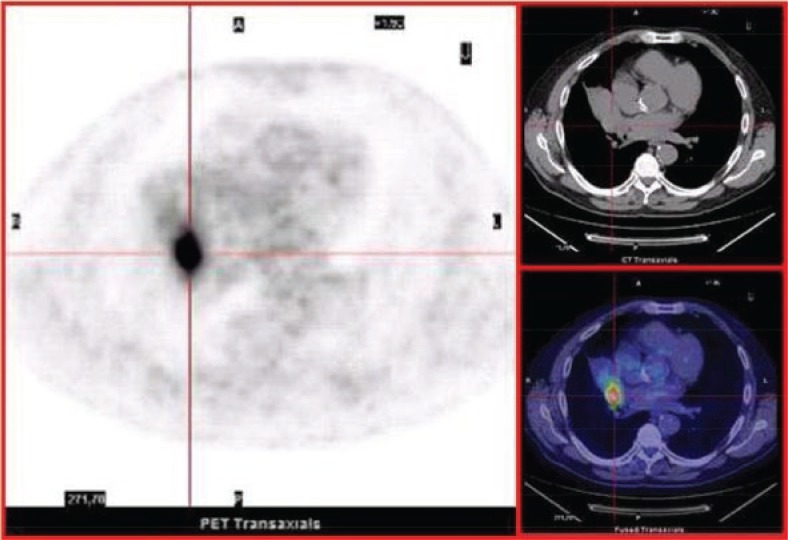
Pre-surgical ^18^F-FDG PET/CT axial images. Abnormal 18F-FDG uptake (SUVmax < 9) in a cancer lesion located in the perihilar region of the right lung (size < 30 mm).
